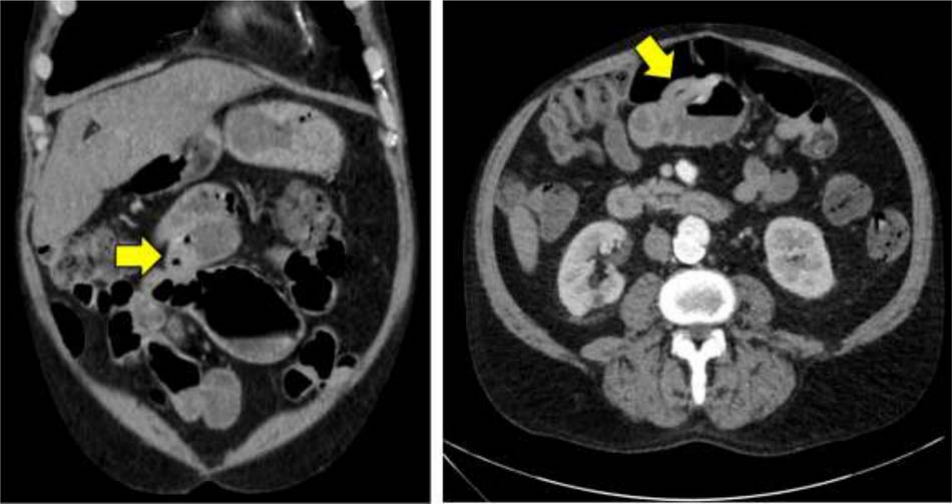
Computed tomography imaging of the abdomen and pelvis showing mild thickening of the wall of the proximal ileum.
